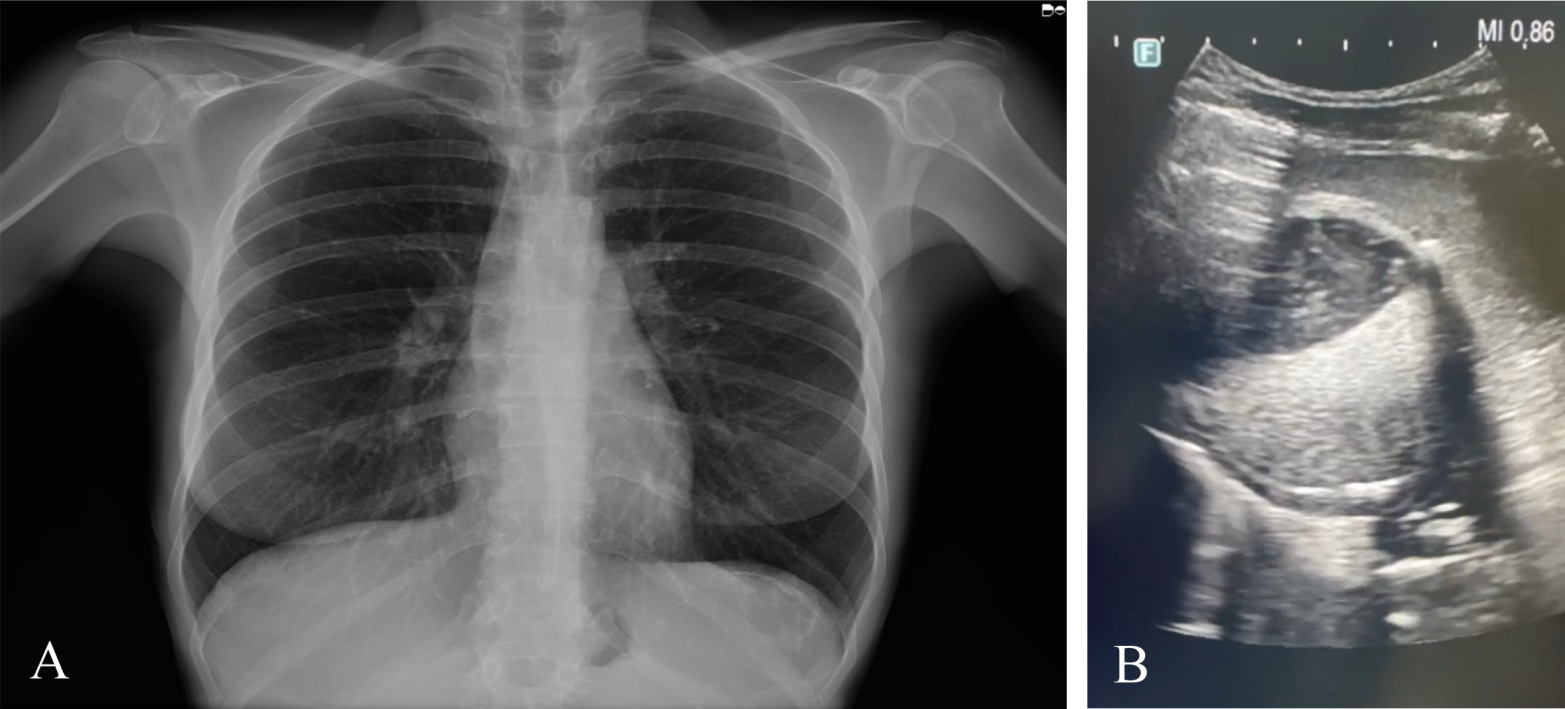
(A) Thoracic conventional radiography displaying an increased volume in the splenic area. (B) Ultrasound evidencing a round-shaped cystic formation in the spleen.
